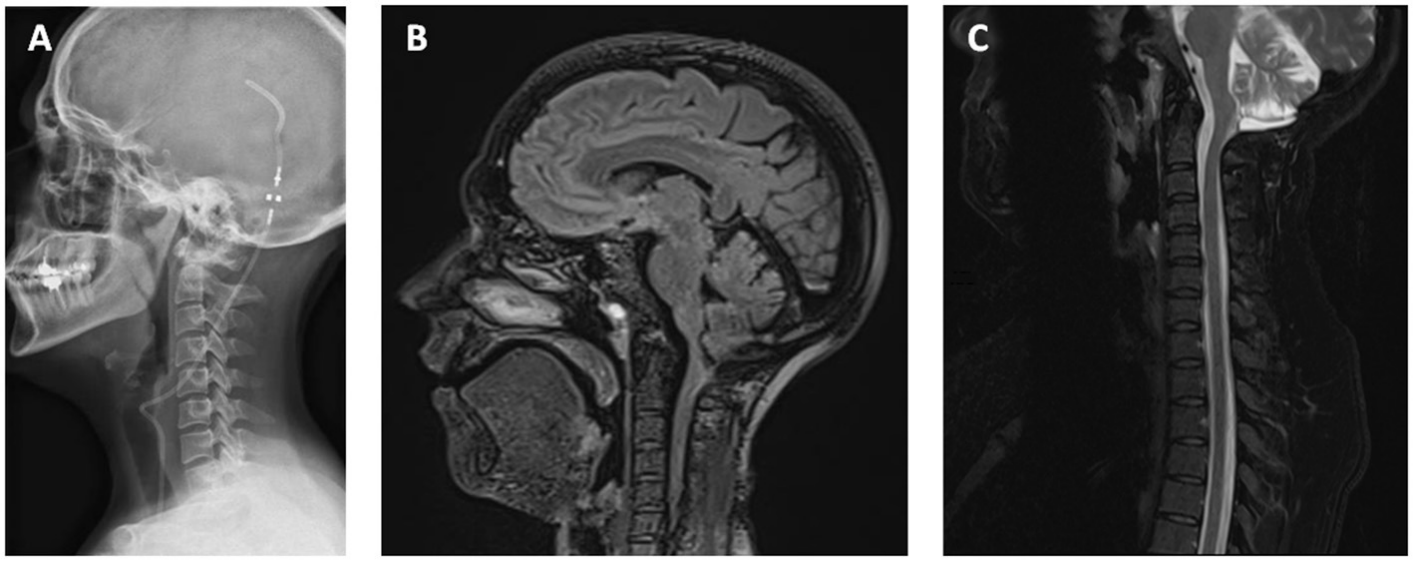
(A) Lateral x-ray showing the ventriculoperitoneal shunt. (B) No hydrocephaly and dilatation of fourth ventricles on MRI sagittal view T1 sequence. The syrinx has completely regressed (C) on MRI sagittal view T2 sequence.
